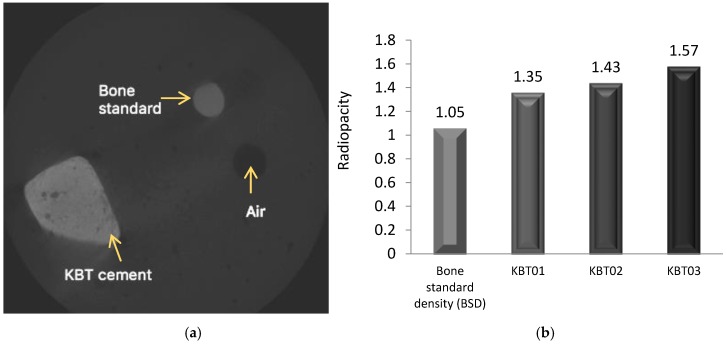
(a) Radiograph image of the cement sample and SP3 standard; (b) Comparison of the radiopacity of the cements.
